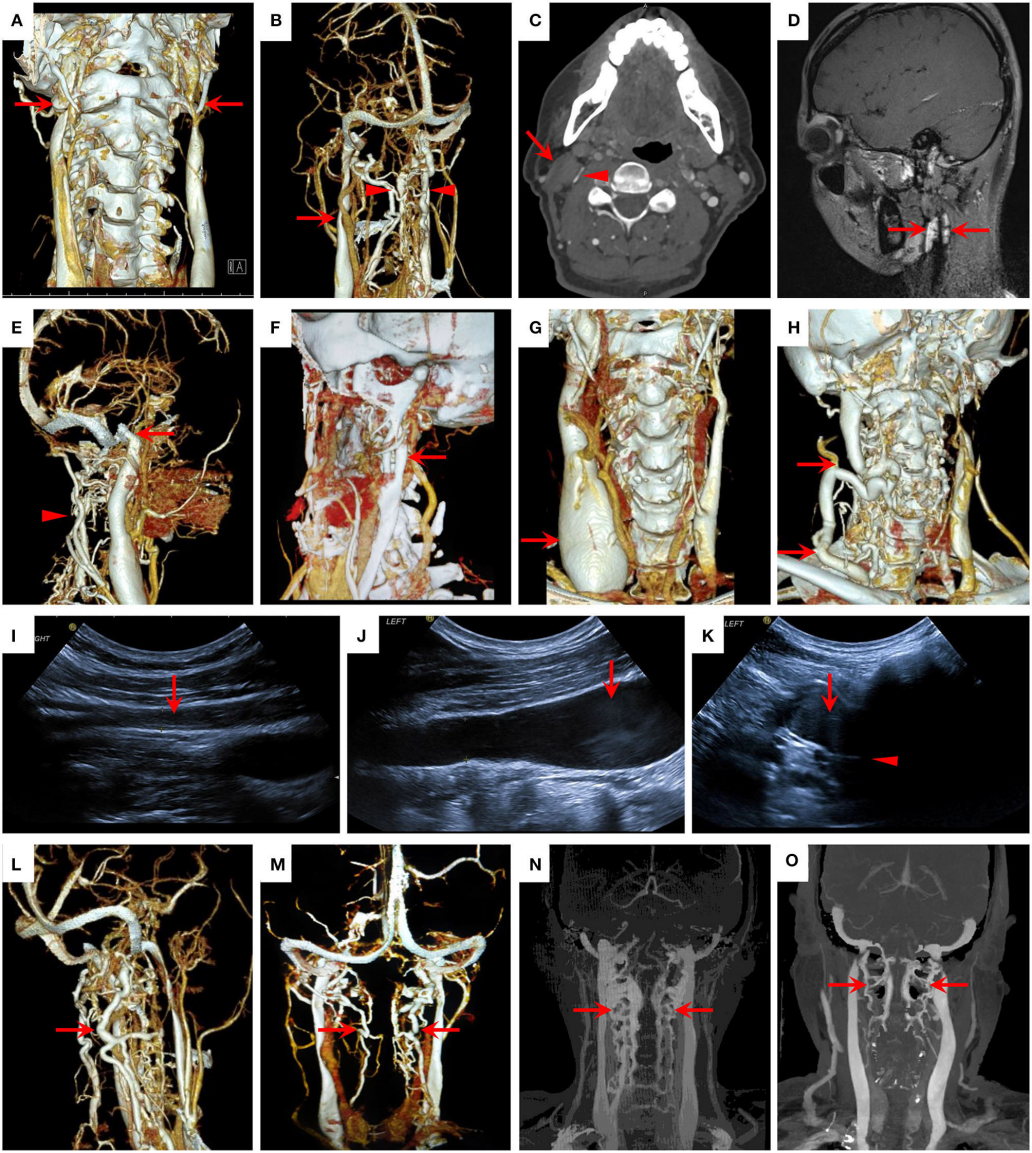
Clinical classification and collateral circulation of CCSVI. (A–D) CCSVI by extra-luminal compression in 3D-computed tomography venography (3D-CTV) and contrast-enhanced magnetic resonance imaging (CE-MRI) maps. (A) Bilateral IJVS compressed by the transverse process of C1 and styloid process at the J3 segments (red arrow); (B) Left IJVS compressed by carotid artery (red arrow) at the J2 segment with abnormally dilated paraspinal collateral veins (red triangle); (C) Right IJVS (red triangle) compressed by sternocleidomastoid muscle (red arrow) at the J2 segment; (D) IJVS compressed by swollen lymph nodes (red arrow) at the J2 segment in CE-MRI. (E–H) CCSVI by malformation in 3D-CTV maps. (E) Right high jugular bulb (red arrow) at the J3 segment with abnormally dilated paraspinal collateral veins (red triangle); (F) Fenestration of left IJV at the J2 segment (red arrow); (G) Right internal jugular phlebectasia at the J1 segment (red arrow); (H) Right tortuous IJV from the J3 to J1 segments (red arrow). (I–K) CCSVI by intraluminal anomaly in jugular ultrasound maps. (I) IJV thrombosis from the J3 to J1 segments (red arrow); (J–K) Elongated venous valve (red triangle) in IJV with erythrocyte aggregation (red arrow) at the J1 segment. (L–O) Degree of vertebral venous expansion in 3D-CTV maps. (L) Severe external vertebral venous expansion (red arrow); (M) Mild external vertebral venous expansion (red arrow); (N) Severe internal vertebral venous expansion (red arrow); (O) Mild internal vertebral venous expansion (red arrow).
